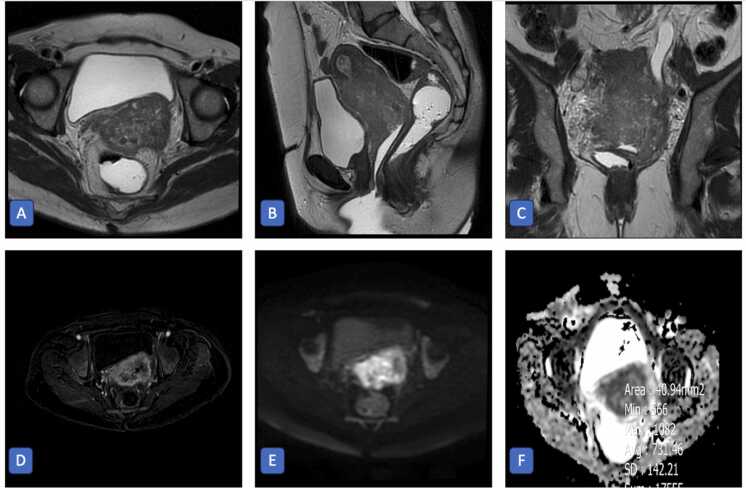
Axial (A), sagittal (B), and coronal (C) T2-weighted, subtraction T1-weighted post-contrast (D), axial diffusion-weighted (E), and ADC (F) MR images of a 57-year-old woman with cervical carcinoma. The tumor measures approximately 76 mm in craniocaudal length and demonstrates parametrial and proximal vaginal invasion, involvement of the left ureterovesical junction with resultant left hydroureteronephrosis, and direct invasion of the adjacent bladder wall. Restricted diffusion with a low ADC value of 731 × 10⁻⁶ mm²/s is observed, consistent with FIGO stage IVA cervical carcinoma.
